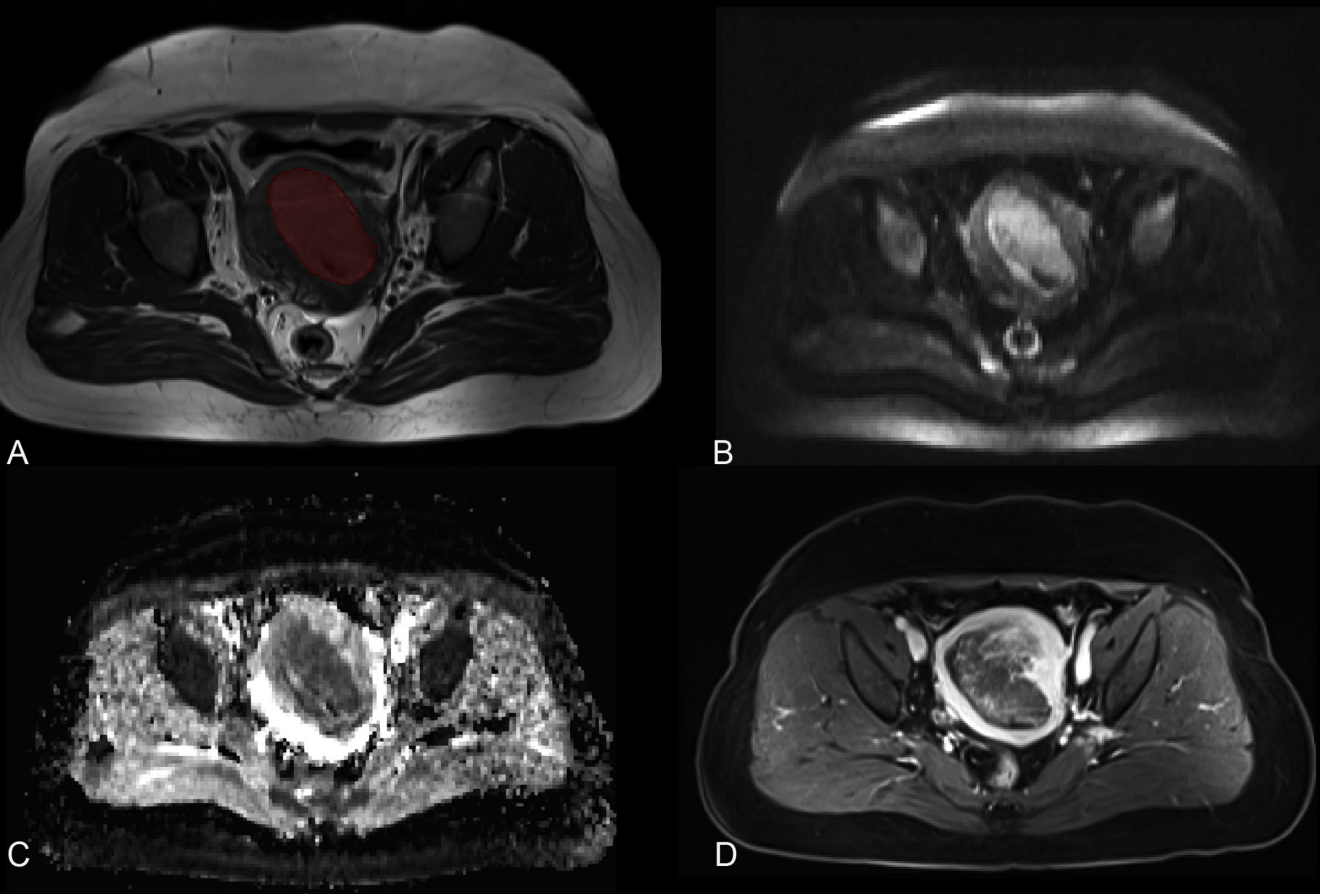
MR images of a 78-year-old woman with endometrial cancer. (A) Axial T2-weighted imaging is marked with a region of interest. (B) Axial diffusion-weighted imaging (b = 800 s/mm^2^). (C) Axial apparent diffusion coefficient imaging. (D) Axial contrast-enhanced T1-weighted imaging.
